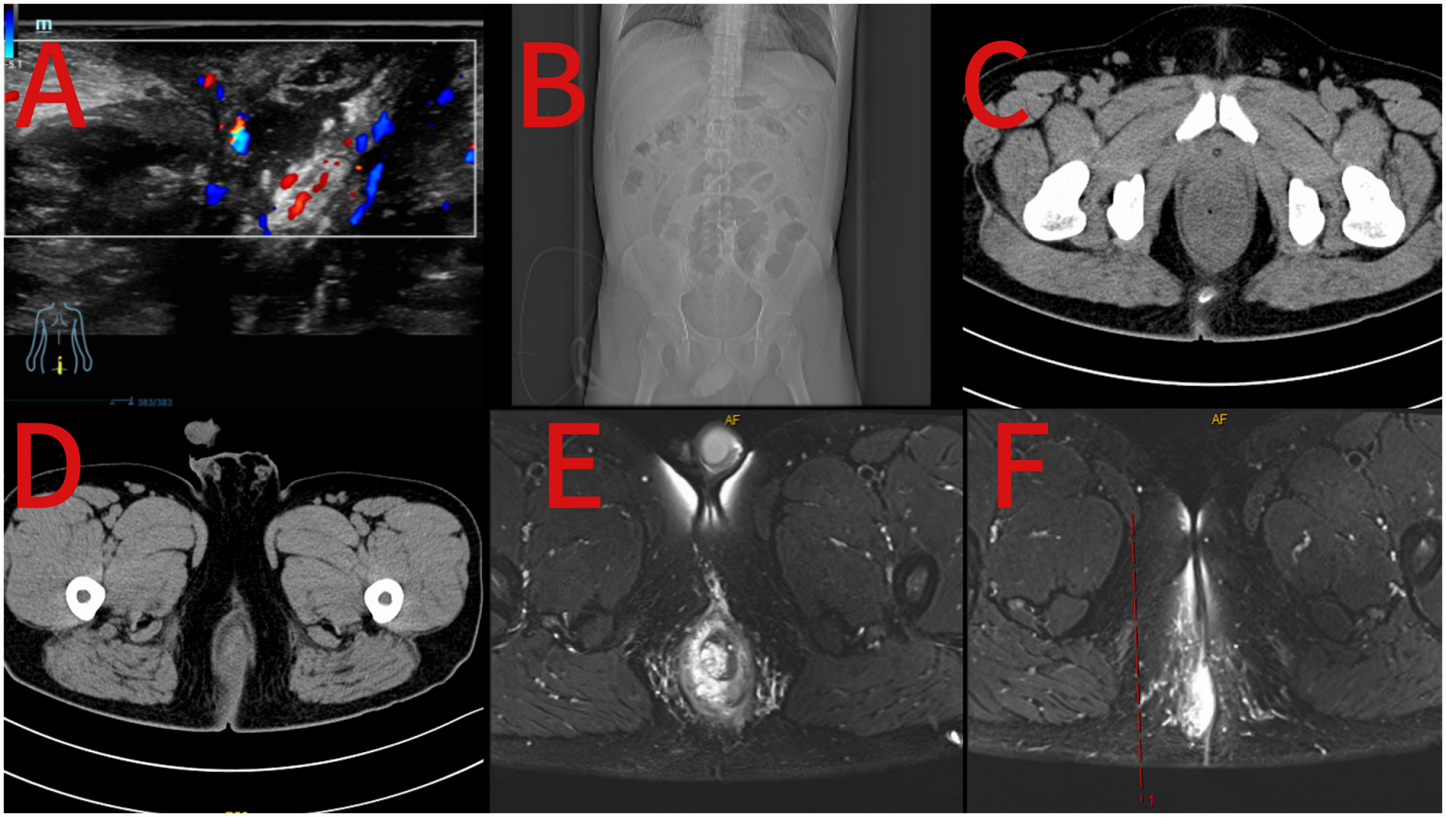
Preoperative ultrasound image of the perianal mass (A); preoperative coronal view of abdominal CT (B); preoperative axial view of abdominal CT (C,D); preoperative 3T MRI images of the anus (E,F).
